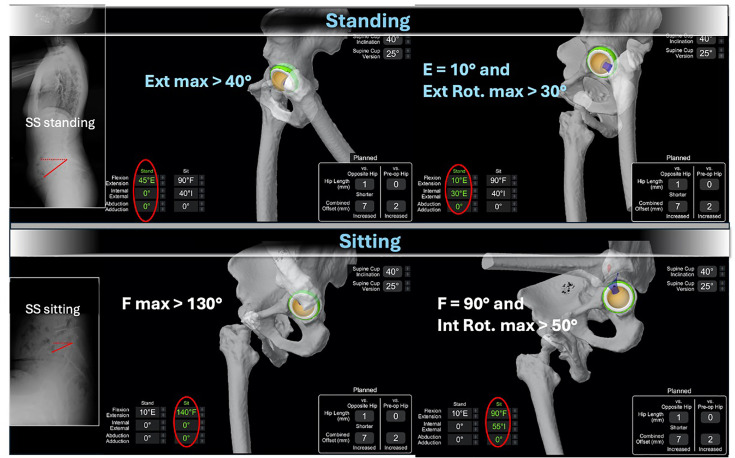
Collision detection model in the V4 software, integrating sacral slope measurements in both standing and sitting positions. The MAKO (Stryker, USA) CT-based planning enables optimization of implant positioning (acetabular component and femoral component) according to functional lumbopelvic kinematics. E, extension; F, flexion; Ext. Rot., external rotation; Int. Rot., internal rotation; SS, sacral slope.
